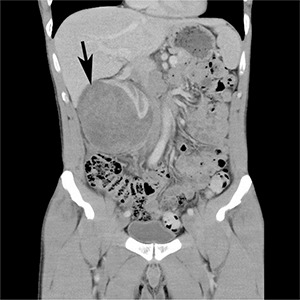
CT scan – coronal view. Large mass lesion (arrow) within right kidney measuring up to 7.4 × 7.5 × 9.2 cm.
